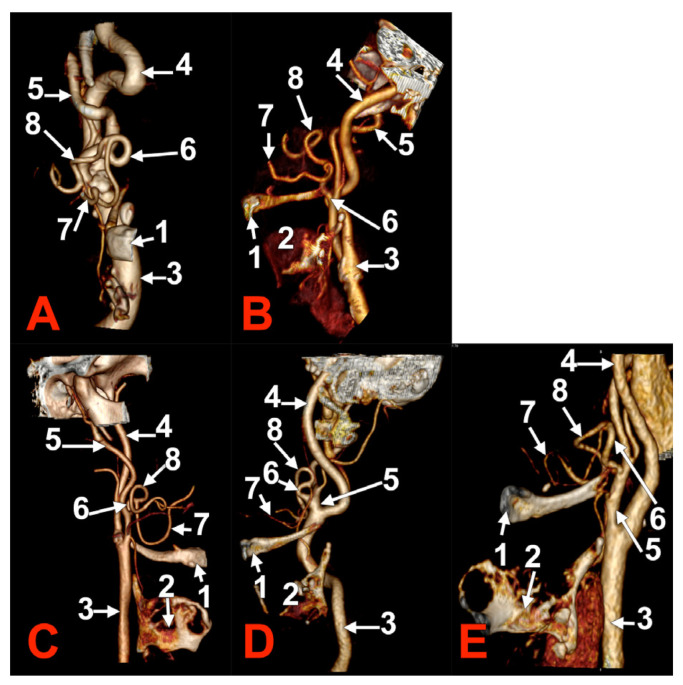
Morphological variants of right unilateral linguofacial trunks. Computed tomography angiograms. Three-dimensional volume renderings. Anterior (A), antero-medial (B,D,E), and medial (C) views. 1. hyoid body; 2. thyroid cartilage; 3. common carotid artery; 4. internal carotid artery; 5. external carotid artery; 6. linguofacial trunk; 7. lingual artery; and 8. facial artery.
